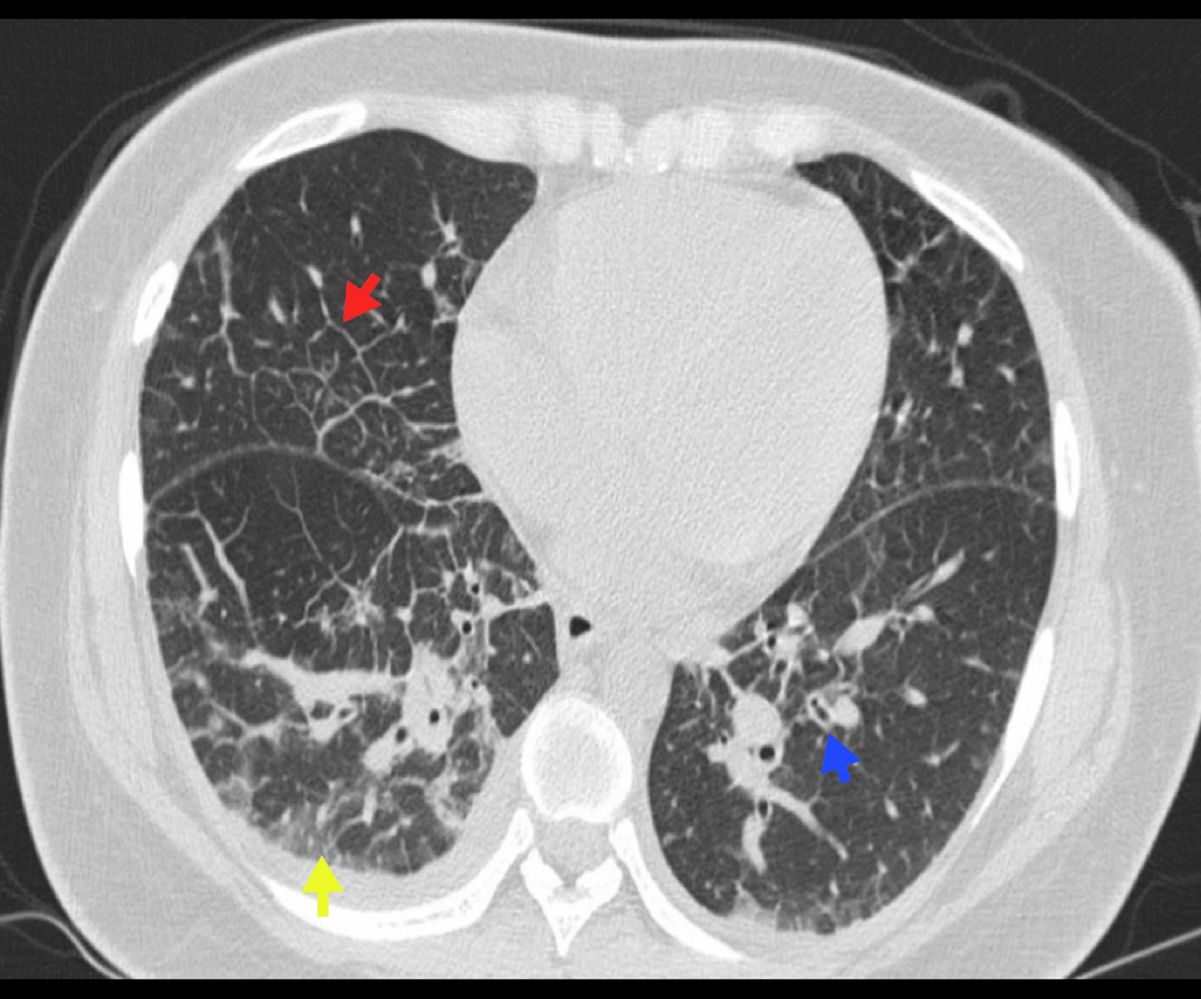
Pulmonary leukostasis A 39-year-old female patient with acute B-cell lymphoblastic leukemia and hyperleukocytosis. Chest CT reveals thickening of the bronchial walls (blue arrow), thickening of the interlobular septa (red arrow), and subpleural ground-glass opacities (yellow arrow). Image courtesy: Pulmonology Service of the Internal Medicine Department, University of Antioquia. Consent for open access publication was obtained from the patient.
